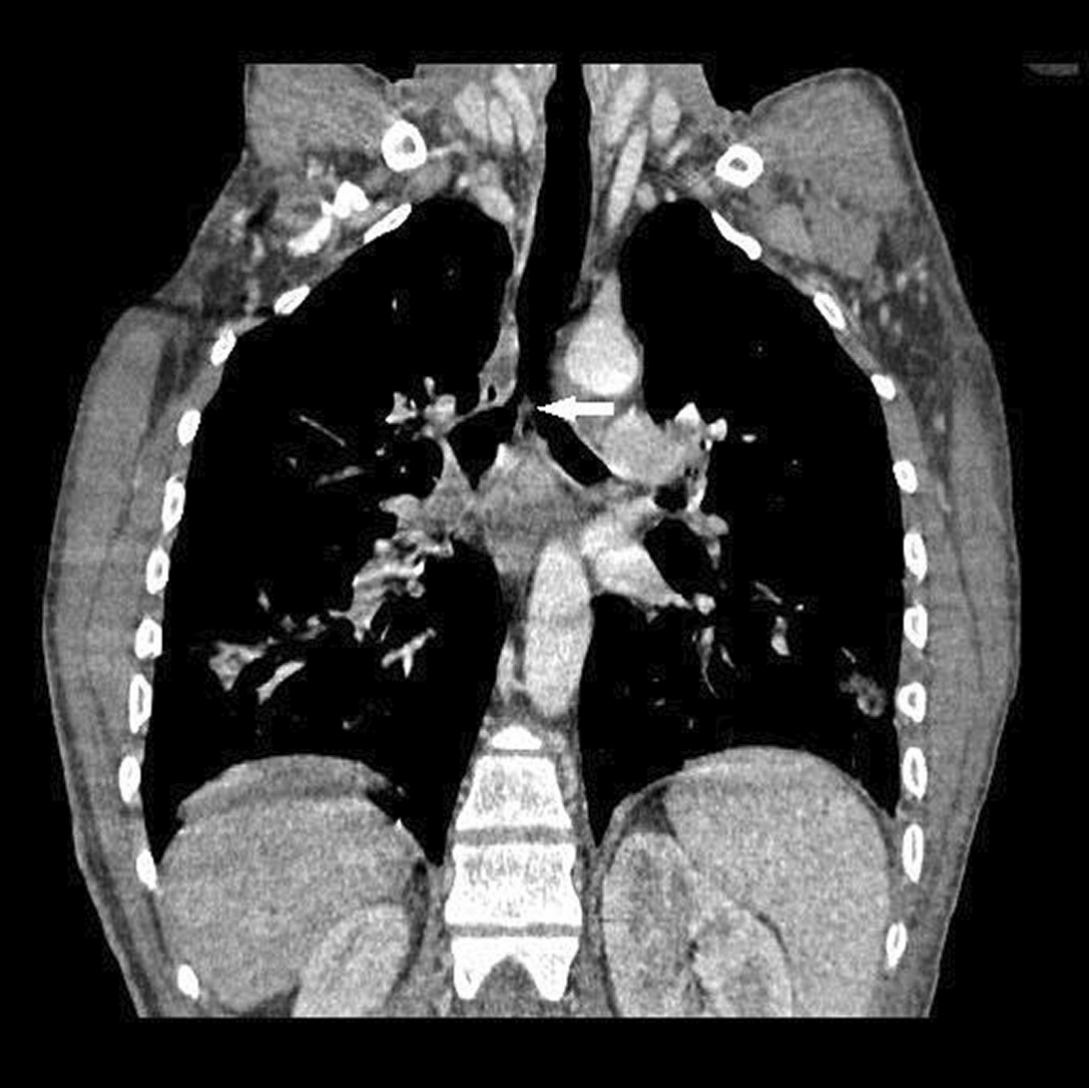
Coronal reconstructed CT image of the chest showing narrowing of distal trachea and main bronchi. A residual fungus mass on the carina (arrow) is seen
